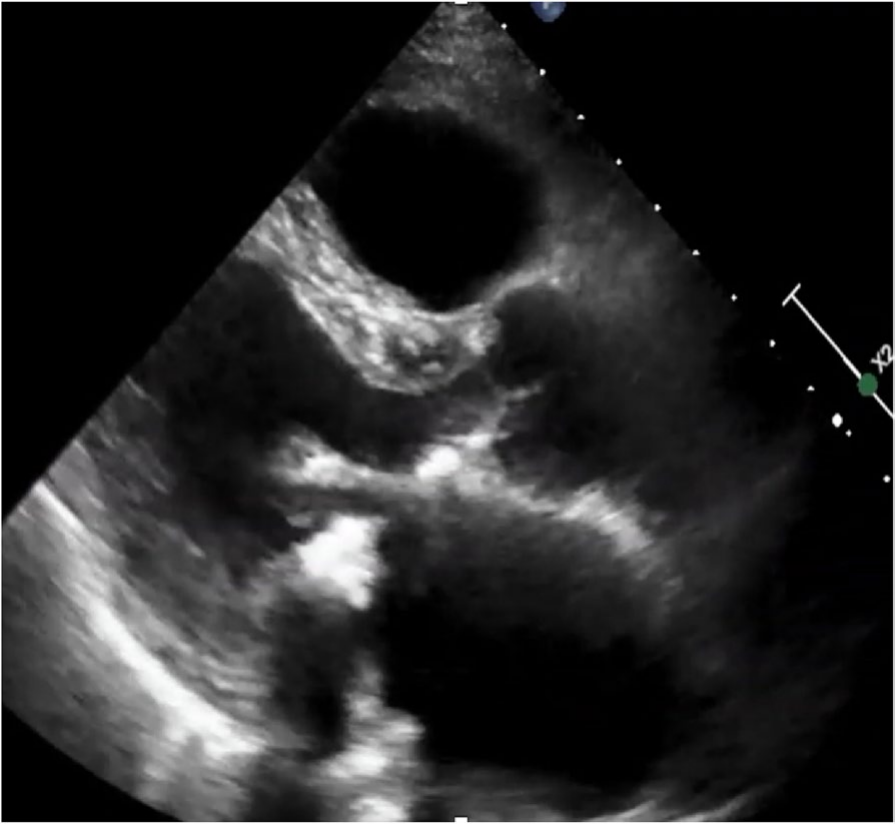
Echocardiography at the initial visit. Transthoracic echocardiography revealed moderate mitral stenosis with bulky mitral annular calcification
